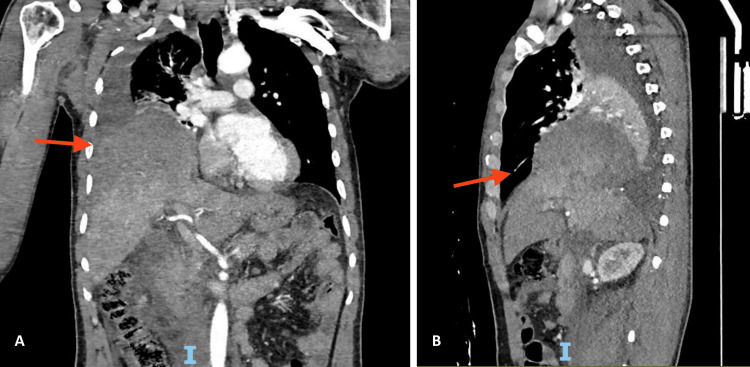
Coronal (A) and sagittal (B) computed tomography scan showing medium-to-large-sized hemothorax compressing the lung, right hemidiaphragm rupture with herniated liver into the right hemithorax, right hepatic lobe parenchymal disruption measuring approximately 12 × 5 cm, and large subcapsular and perihepatic hematoma representing grade 4 liver injury.
